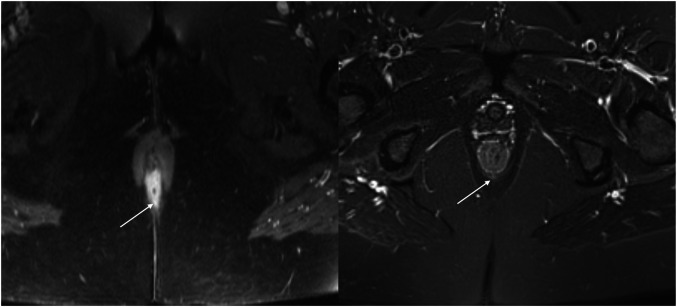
TOpClass–radiological improvement (not complete TOpClass-RH) with majority agreement for not healed. From left to right: Baseline MRI (left): Axial oblique fat-saturated T1-weighted image demonstrates an active fistula at the 6 o’clock position. Follow-up MRI (right): Axial oblique fat-saturated T2-weighted image demonstrates interval improvement but persistent subtle hyperintensity within the posterior anal sphincter (arrow), resulting in classification as not meeting TOpClass radiological healing criteria (2:1 reader agreement)
